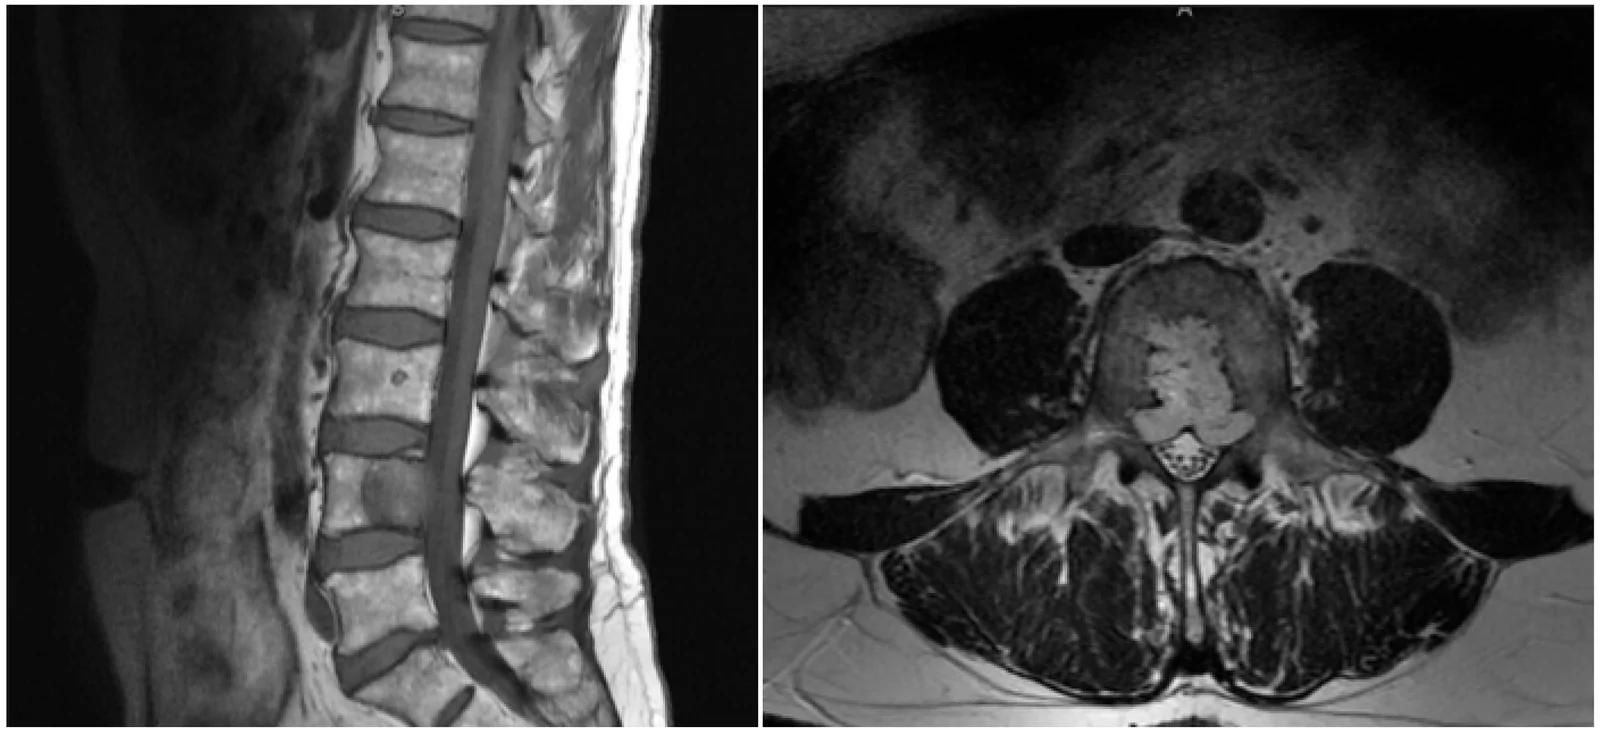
Preoperative MRI Lumbar Spine Sagittal and coronal planes.
